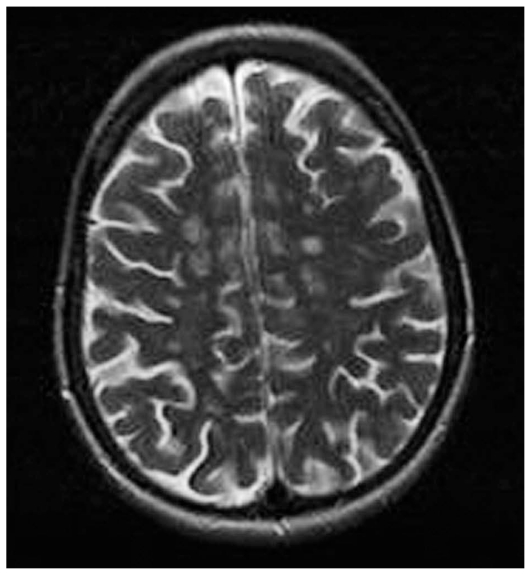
Brain MRI in multiple sclerosis shows multiple lesions (hyper-intense on T2-weighted images) located in the white matter of both hemispheres.
